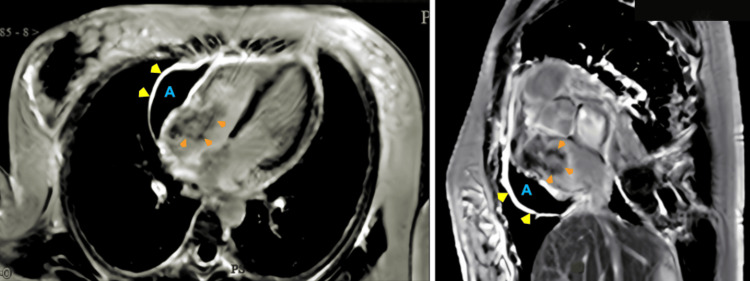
Cardiac MRI, axial and sagittal planes. The pericardial membrane (yellow arrowheads) was thick and enhanced with notable pericardial effusion (letter A). (Left panel) After gadolinium administration, the mass (orange arrowheads) demonstrated hyperenhancement features. (Right panel) Sagittal plane, a significant portion of the mass (orange arrowheads) is located in the RA, with many features suggesting an underlying malignant pathology, including thick pericardium (yellow arrowheads) and pericardial effusion (letter A). MRI, magnetic resonance imaging; RA, right atrium
